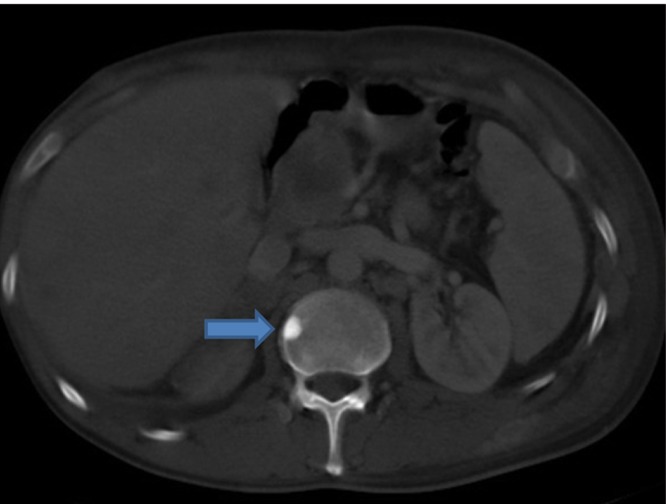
G, Abdominal CT with bone window shows sclerotic metastasis in the vertebral body (blue arrow).
